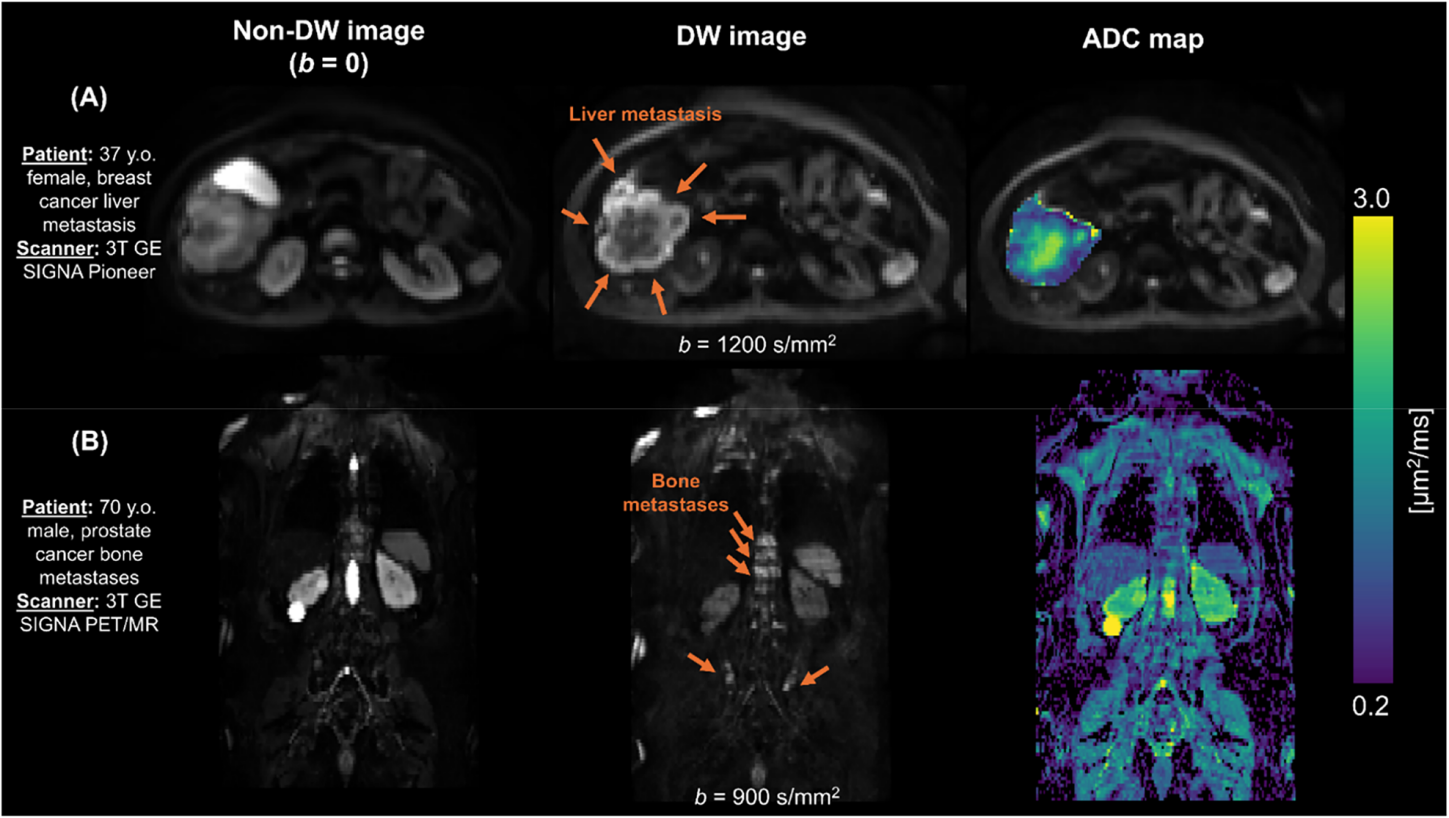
Examples of DW images and ADC maps in body tumours. From left to right: non-DW image (*b* = 0); DW image; ADC map. A 37-year-old breast cancer female patient, presenting with a liver metastasis, was scanned on a 3 T GE SIGNA Pioneer system. B 70-year-old prostate cancer male patient, presenting with disseminated bone metastases. The figure demonstrates the unique ability of DWI to highlight between- and within-tumour contrasts, e.g., intense DW signal attenuation (and hence high ADC) in the core of the breast cancer liver metastasis, compatible with the presence of a tumour necrotic core. Images courtesy of Raquel Perez-Lopez, Francesco Grussu, Alonso Garcia-Ruiz (VHIO, Barcelona, Spain)
